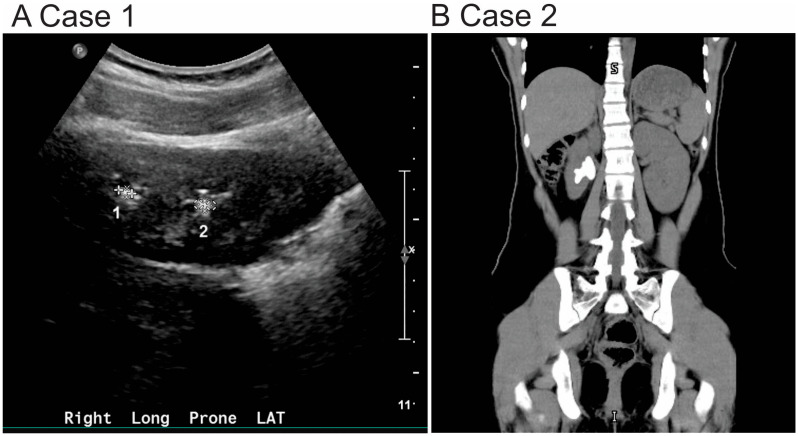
Radiographic findings in two pediatric patients diagnosed with cystinuria. (A) Renal ultrasound of Case 1: The kidneys are normal in size and position. In the right kidney, there are subcentimeter calculi at the upper and lower poles, each measuring up to 4 mm (indicated as 1 and 2). In the left kidney, a 3 mm nonobstructing calculus is seen at the lower pole (data not shown). No hydronephrosis or hydroureter is present. (B) Non-contrast CT of the abdomen and pelvis (Case 2): A 3.5 cm staghorn calculus is seen extending from the mid to lower pole of the right kidney into the renal pelvis. No ureteral or left-sided calculi are identified.
